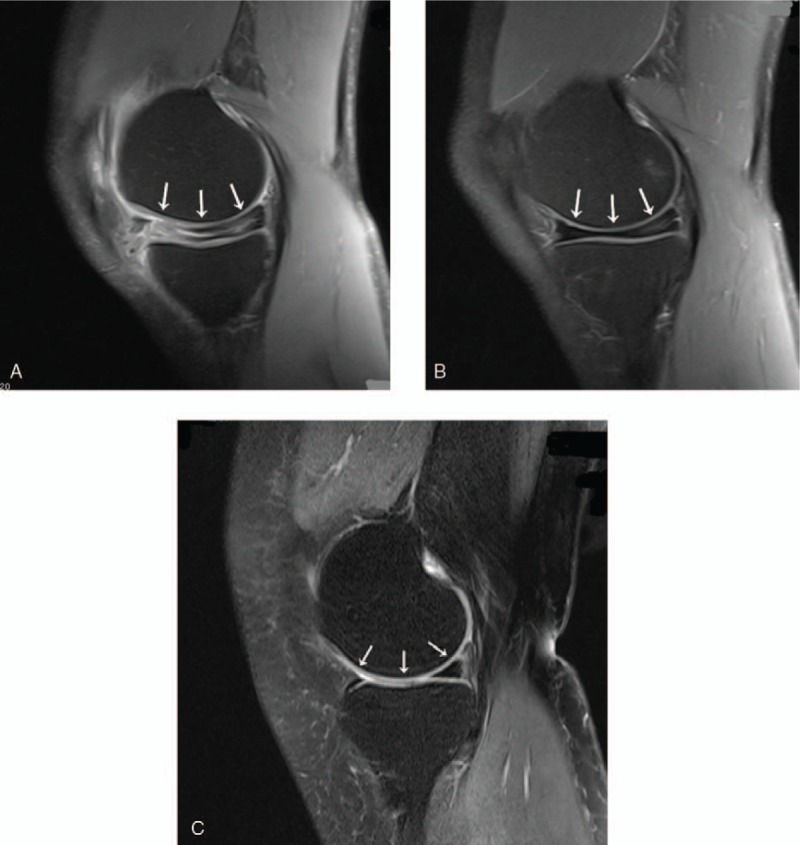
MRI images of the patient (A, B) and a normal knee (C) in the sagittal plane. (A) T2-weighted sagittal MRI of the left knee showing the discoid medial meniscus with a horizontal and bucket-handle-like tear. (B) Discoid medial meniscus with grade II injury in the right knee. (C) Normal medial meniscus. (A, B) The distal and posterior sections of the femoral medial condyles were more flattened (indicated by the white arrows) than normal (C). MRI = magnetic resonance imaging.
